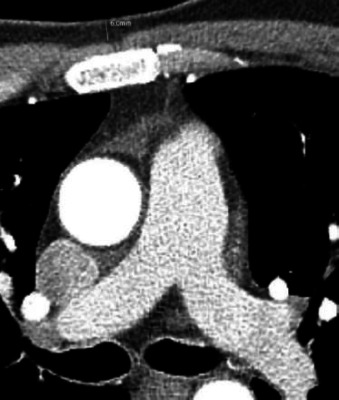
Presternal fat thickness was measured from anterior to posterior at the level of the pulmonary artery bifurcation on axial CT image.
